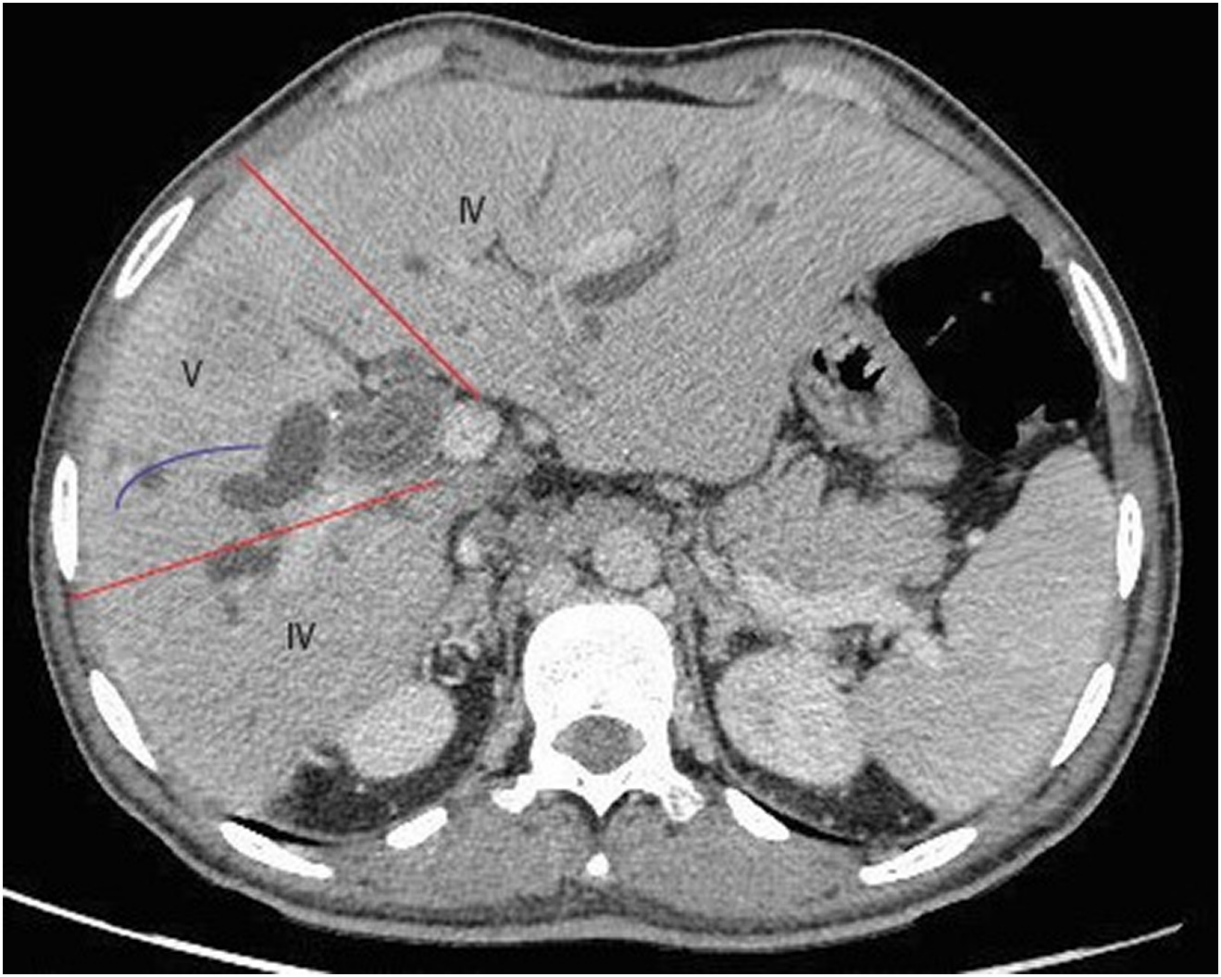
Segment 5 in abdominal axial CT SCAN showed a lobulated lesion (blue arrow). The lesion communicated with the dilated right hepatic biliary tree, and thin septations and dense material were observed within the dilated biliary tree, indicative of an intrahepatic biliary tree‐ruptured hydatid cyst.
